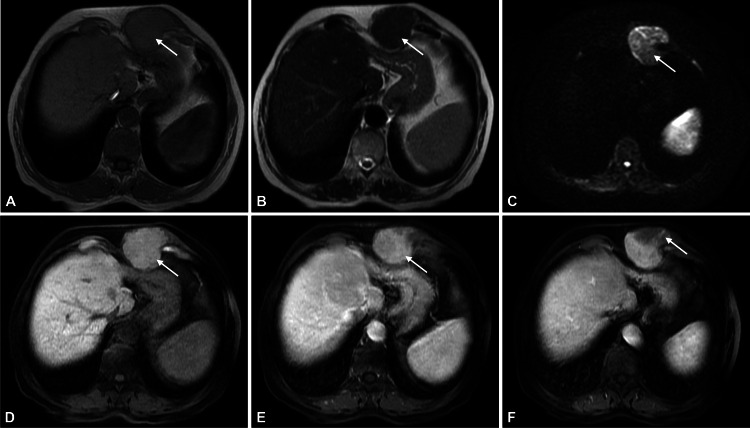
Abdominal MRI Axial T1-WI (A), axial T2-WI (B), axial DWI 600 sec/mm2 (C), axial pre- (D) and post-contrast fat-suppressed 3D-GRE T1-WI in the arterial (E) and portal-venous (F) phases. A round 6 cm mass is depicted at the anterior abdominal wall, showing T1-WI (arrow, A) and T2-WI (arrow, B) signal intensity similar to the liver parenchyma, which favors hepatocellular nature. On DWI, the mass is hyperintense on the higher gradient (b = 600 sec/mm2) (arrow, C), increasing the likelihood of malignancy. In a dynamic contrast-enhanced study, the lesion shows iso-enhancement with the liver during the arterial phase (arrow, E). However, there are small areas of washout seen in later phases (arrow, F). MRI: magnetic resonance imaging, WI: weighted images, DWI: diffusion-weighted images
